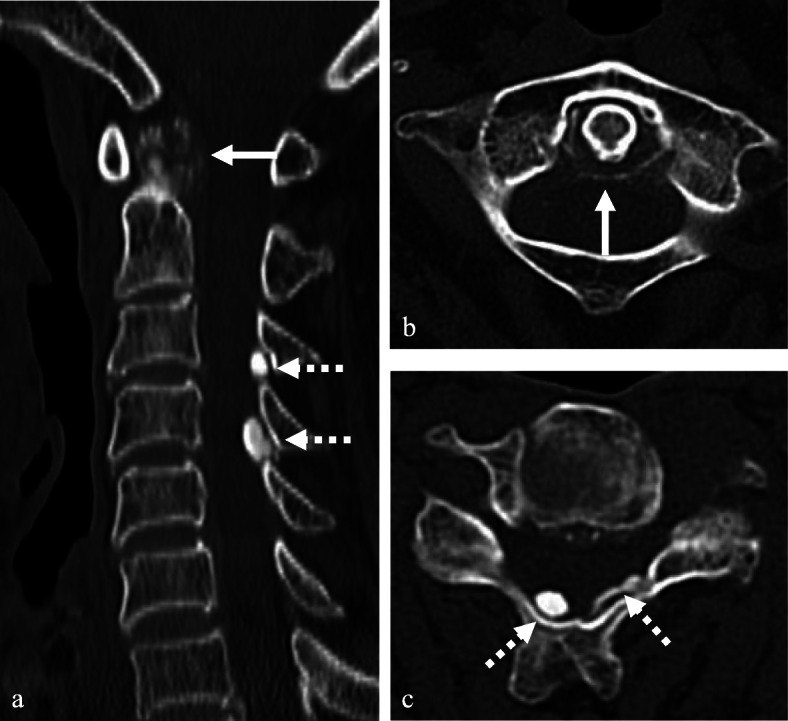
Sagittal and axial CT scans showed a calcified lesion behind the dens (a and b) (white arrows) and spinal cord compression by calcified round lesions at C3 and C4 (a and c) (dotted white arrows)
